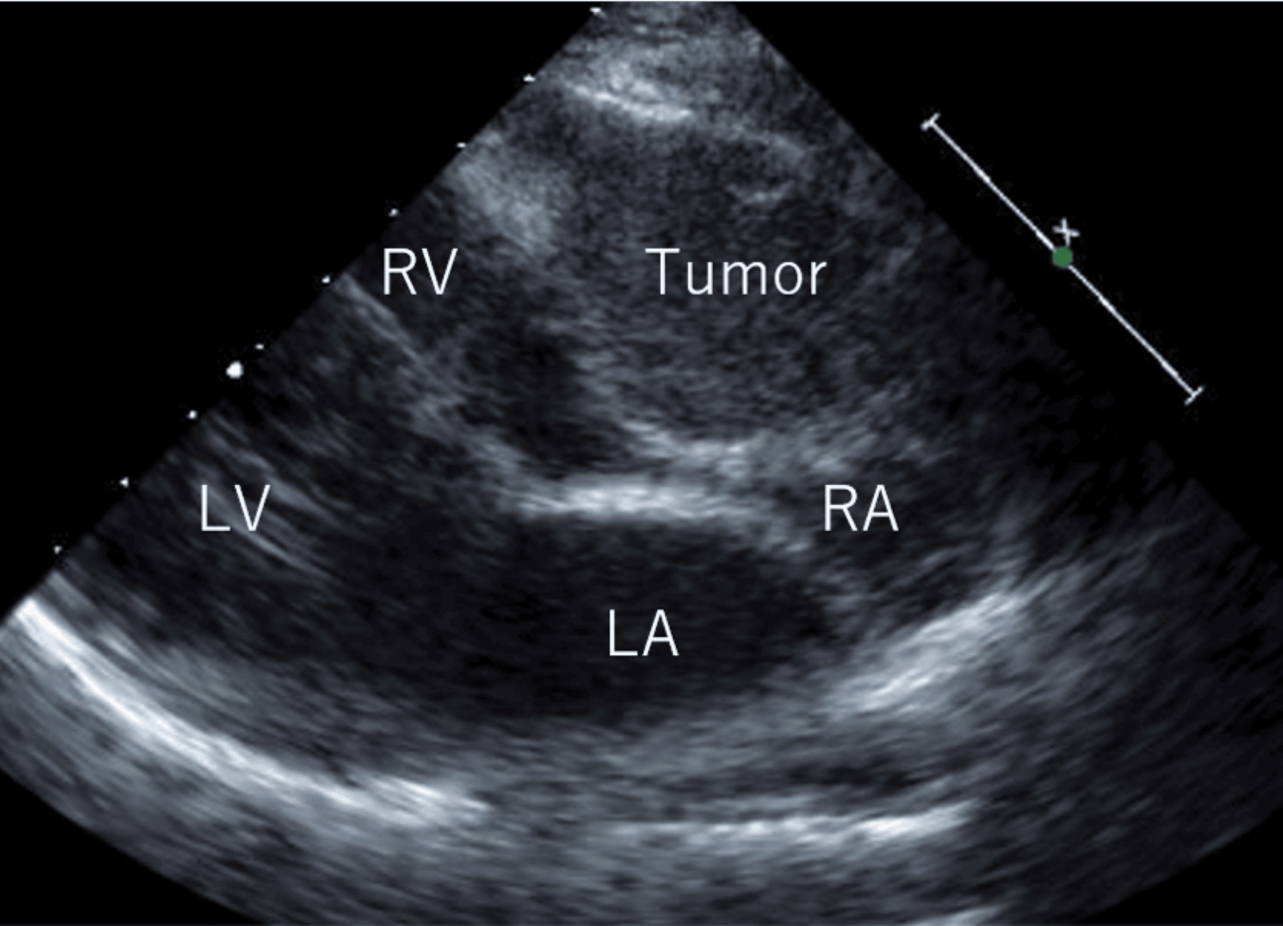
Preoperative echocardiography. Echocardiography revealed abnormal structures in the right atrium. RA: right atrium; LA: left atrium; LV: left ventricle; RV; right ventricle
